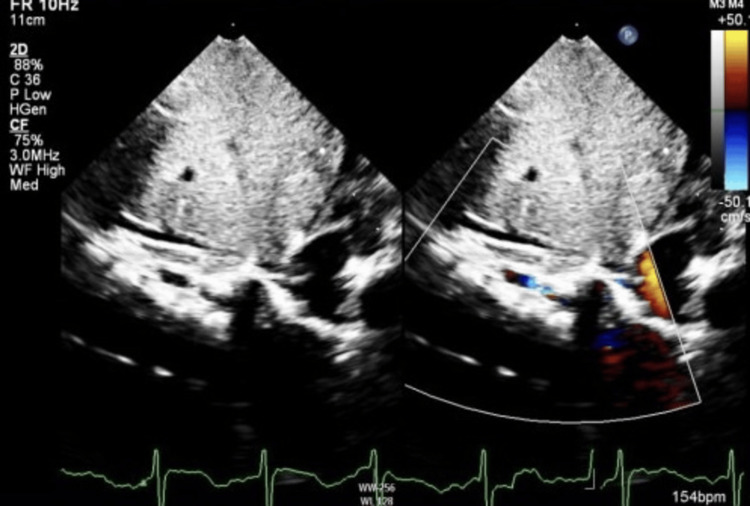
Subcostal view of transthoracic echocardiography with two-dimensional color Doppler demonstrating the anomalous right pulmonary vein drainage into the IVC IVC: inferior vena cava
